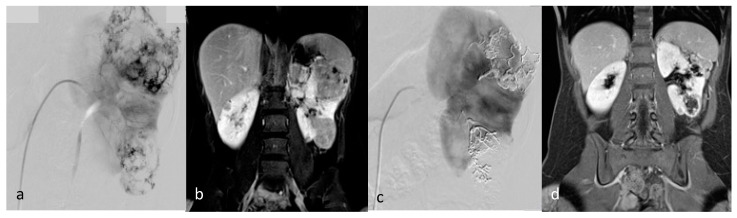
Selective arterial embolization (TAE) of two large left AML. (a) Angiography shows two AML with dysplasic vascularization. (b) MRI showing the two AML on coronal T1 FatSat VIBE Sequence with Gadolinium with hyposignal T1 aneurysms before TAE. (c) Final angiographic control after TAE of the two arterial branches with ONYX 18 (6% EVOH) with technical success (lack of opacification of the AML) (d) MRI showing the two AML on coronal T1 FatSat VIBE Sequence with Gadolinium after TAE, with a volume shrinkage of 83 % for the upper one and 49% for the lower one.
